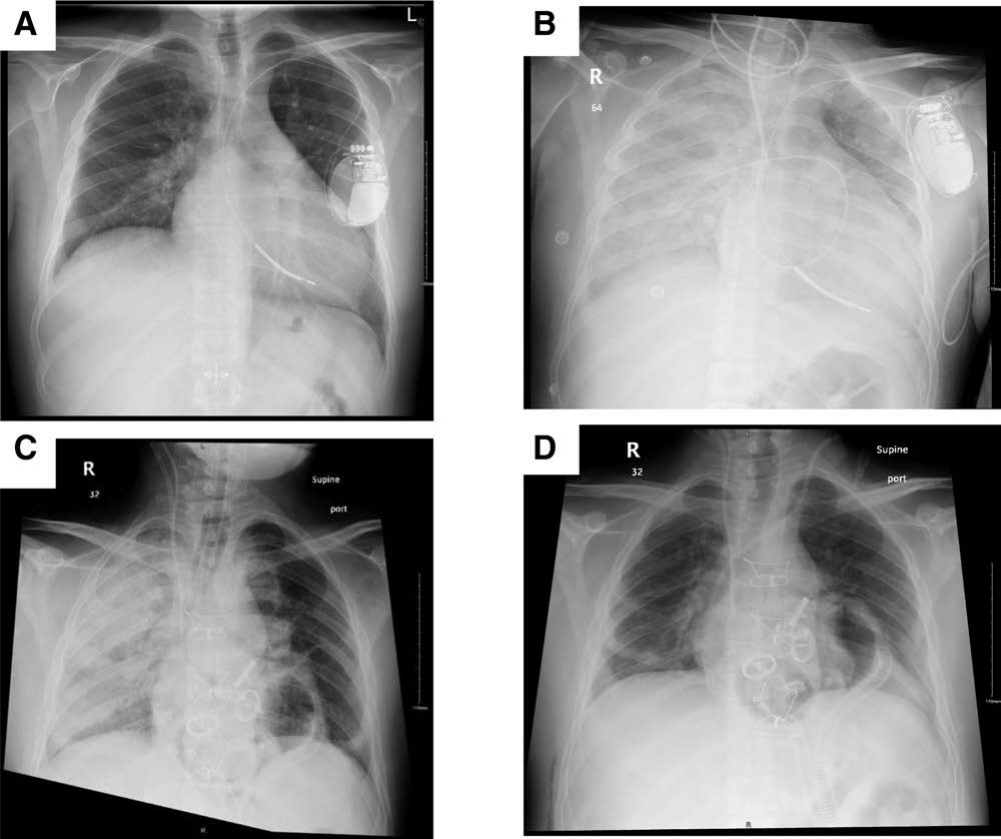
The most relevant chest X-rays during his course of management; (A) Chest X-ray on the day of admission, d 1, February 25, 2023. (B) Chest X-ray just prior to TAH implantation while the patient was on V-A ECMO, d 14, March 10, 2023. (C) Chest X-ray day post TAH implantation, d 15, March 11, 2023. (D) Chest X-ray 1 wk after initiation of Paxlovid therapy; d 24, March 20, 2023.
